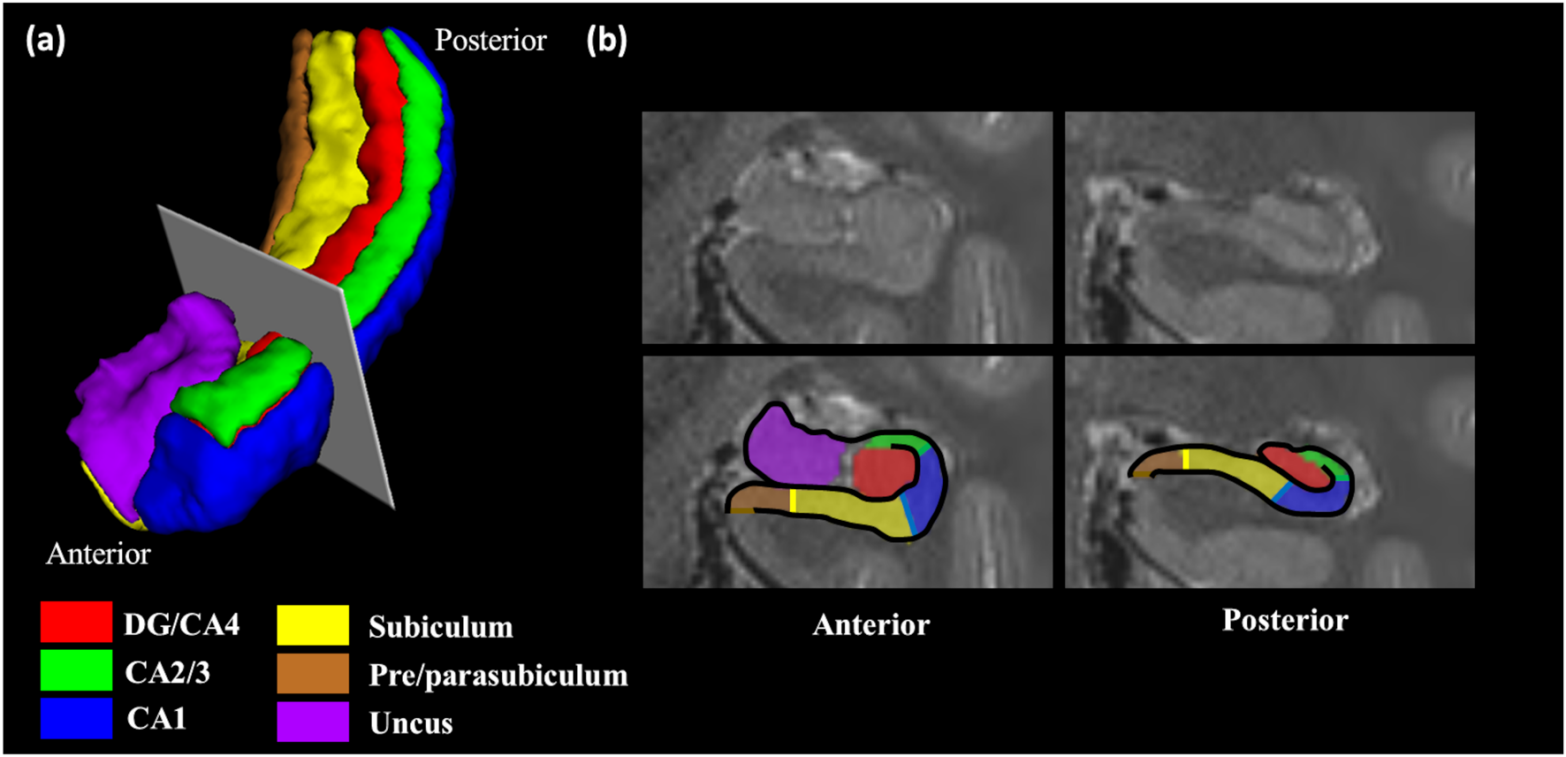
The hippocampal subregions examined in this study. (a) A 3D representation, viewed from an antero-lateral perspective, of the segmented hippocampal subfields, and the boundary used to divide the anterior and posterior portions. (b) Two sections from T2-weighted structural MRI scans of an example hippocampus (top panel) overlaid with hippocampal subfield masks (lower panel). The left image is from the anterior hippocampus, and the right image from the posterior hippocampus.
